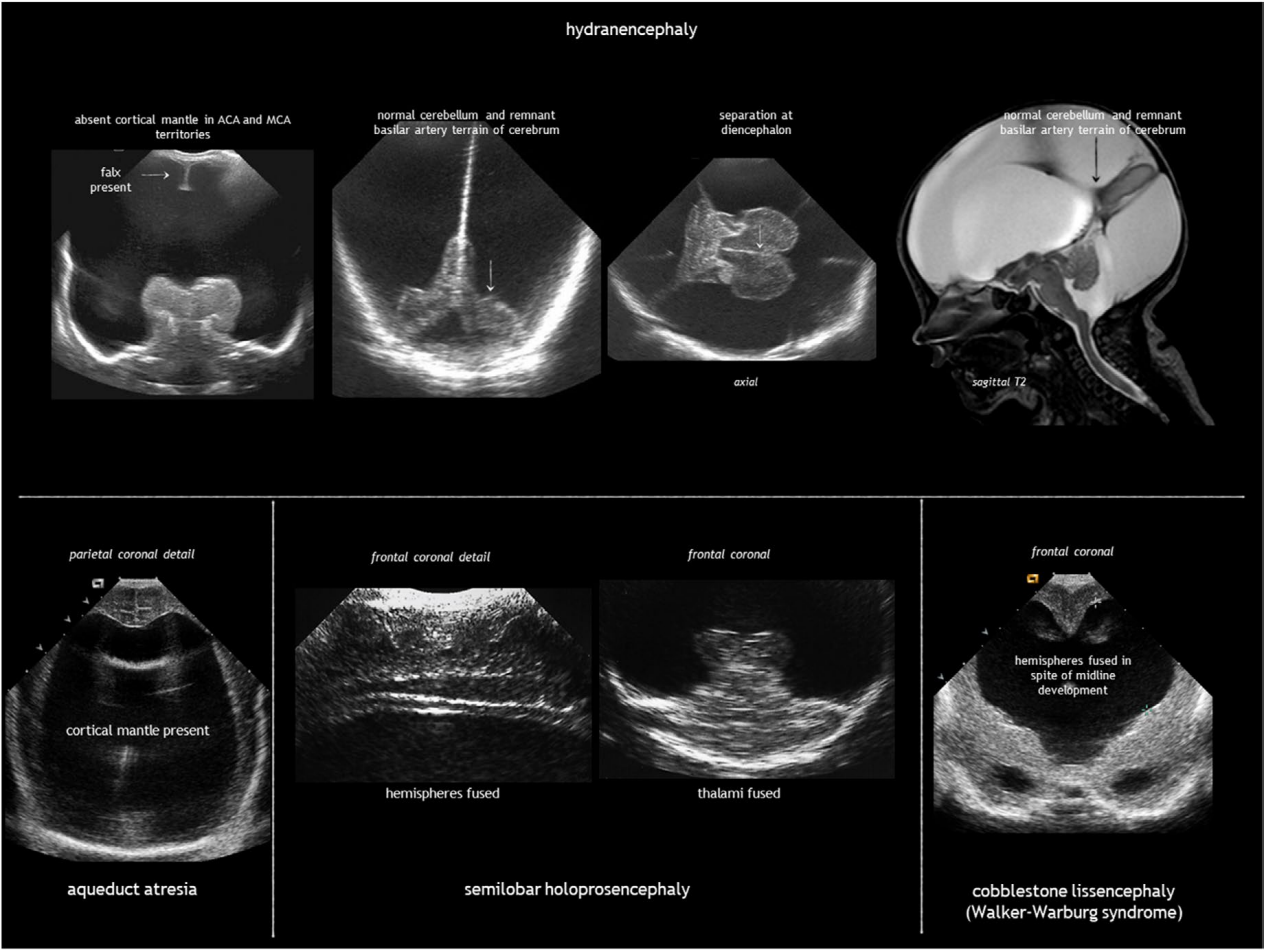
Hydranencephaly and its differential diagnosis on cranial ultrasound. Abbreviations: ACA, anterior cerebral artery; MCA, middle cerebral artery.
